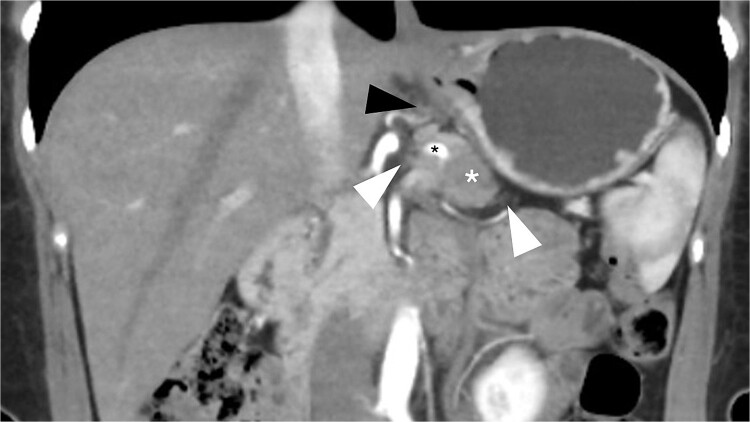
Preoperative CT scan (coronal view, portal phase) shows a 25 mm spiculated mass with hypoattenuation observed after the administration of IV contrast (white asterisk). The lesion encases the splenic artery (black asterisk), extends to the retroperitoneal fat (white arrowhead), and shows mesogastric distortion (black arrowhead).
